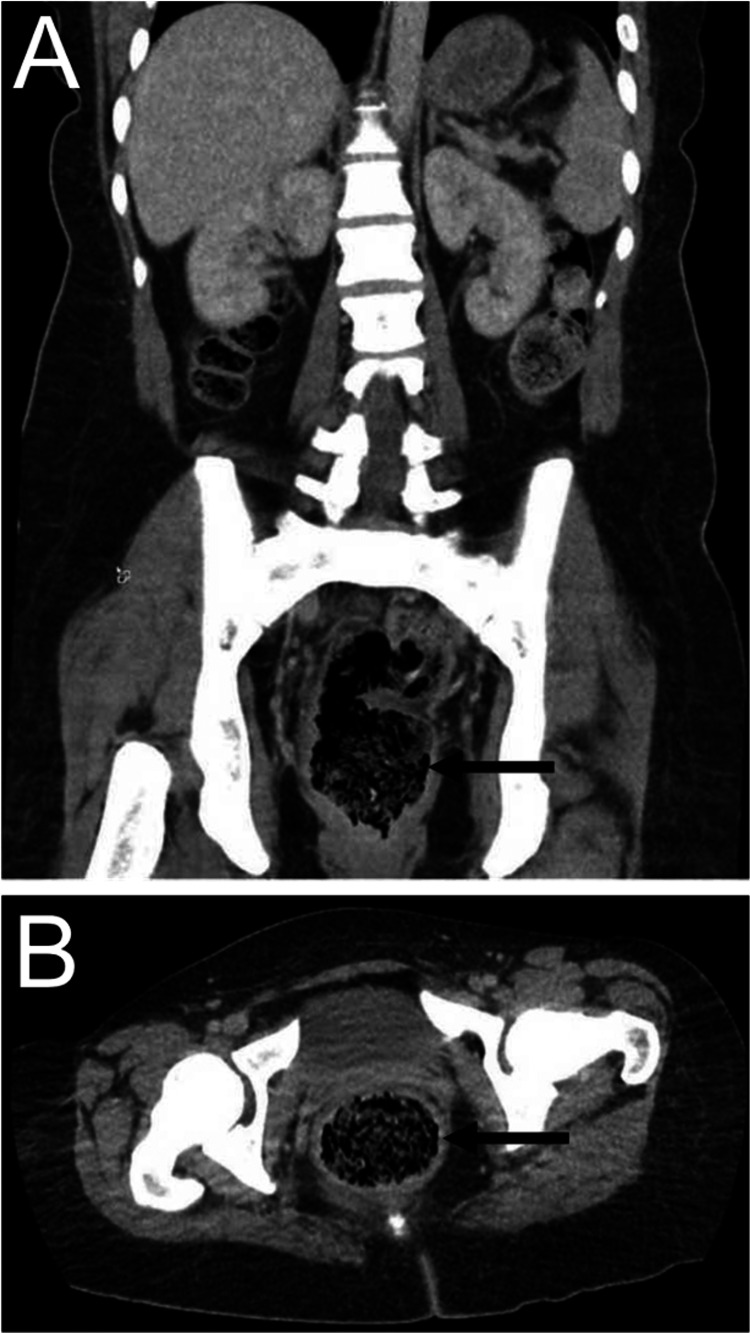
Coronal (A) and axial (B) sections of the CT abdomen and pelvis. Arrows indicate fecal impaction in the rectum.
